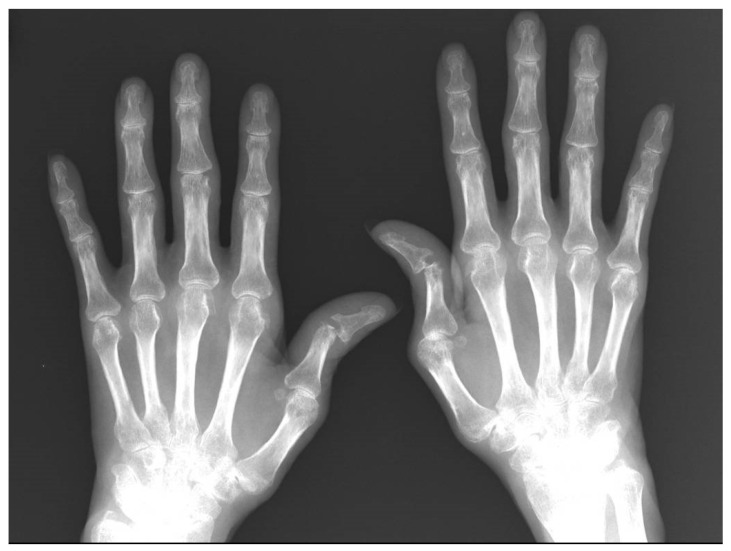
Anteroposterior hand X-ray with subluxation of the metacarpophalangeal and interphalangeal joints of first fingers, and erosions in metacarpal heads and proximal interphalangeal heads.
